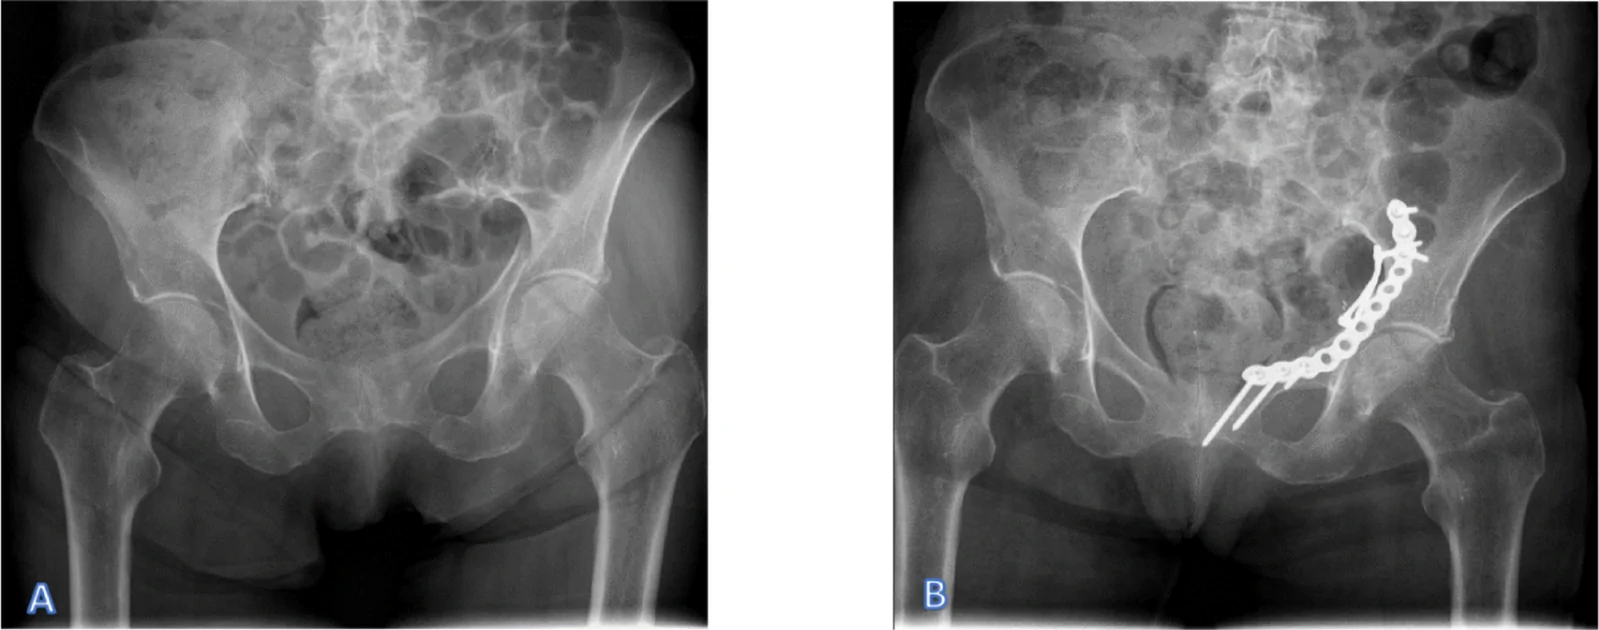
X-ray images extracted from the hospital’s radiology system (Philips IntelliSpace PACS) representing the ORIF treatment: A Preoperative x-ray imaging; B Postoperative x-ray imaging after ORIF
